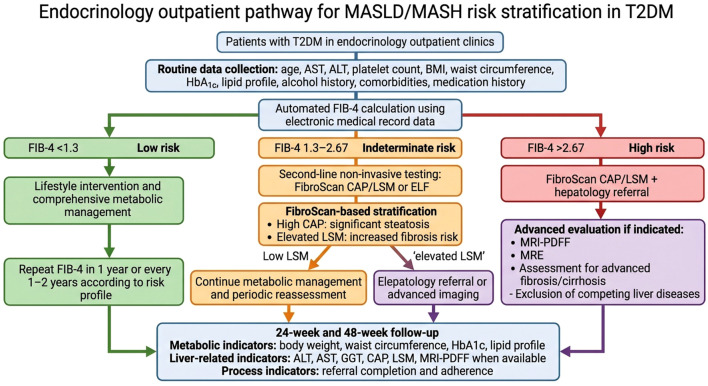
Proposed endocrinology outpatient pathway for MASLD/MASH risk stratification in patients with T2DM. Patients with T2DM undergo routine data collection and automated FIB-4 calculation, followed by low-, indeterminate- or high-risk stratification. Low-risk patients remain in endocrinology-led metabolic management with periodic reassessment; indeterminate-risk patients undergo FibroScan CAP/LSM or ELF testing; and high-risk patients require hepatology referral, with MRI-PDFF or MRE considered when clinically indicated. The FIB-4 and LSM thresholds shown in the pathway should be interpreted as pragmatic triage thresholds derived from current guidance, not as locally validated cut-offs for every population. The pathway incorporates 24-week and 48-week follow-up to assess metabolic indicators, liver-related indicators, referral completion and adherence.
